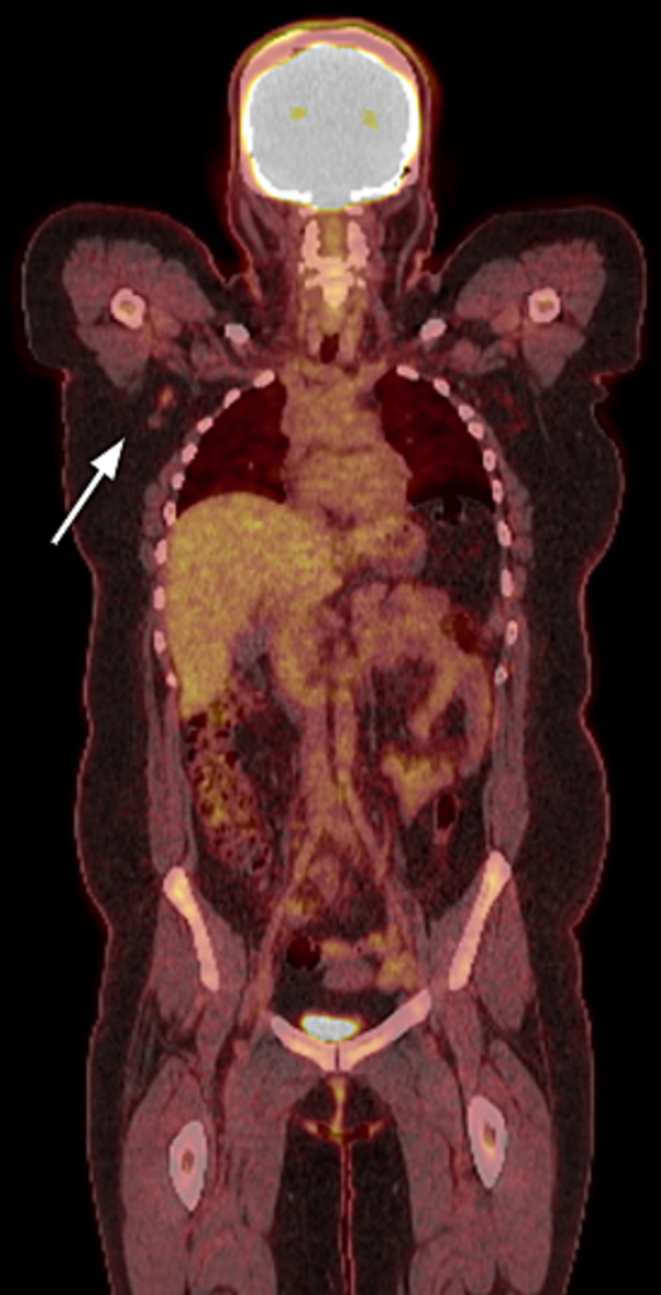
PET-CT scan of the patient in April 2022 demonstrating positive scan for a right sided axillary lymph node.
